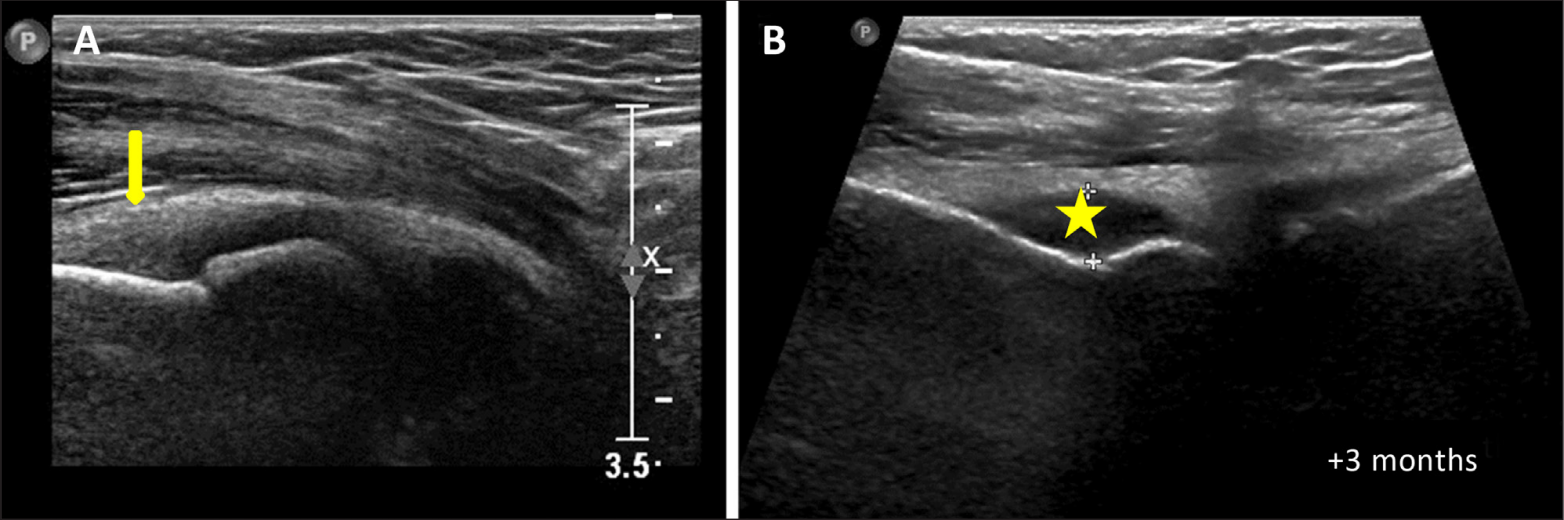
Three months later, as the symptoms persisted, a new ultrasound of the elbow was performed, demonstrating joint effusion (asterisk on Figure 1B); elbow radiograph on the same day showed metaphyseal sclerosis (blue asterisk on Figure 2A), peri-articular osteopenia (green asterisk on Figure 2A) and periosteal apposition (arrows on Figure 2A). Septic arthritis was suspected and a joint lavage was performed; bacteriological samples were negative. Treatment with ledertrexate for chronic synovitis was started.
